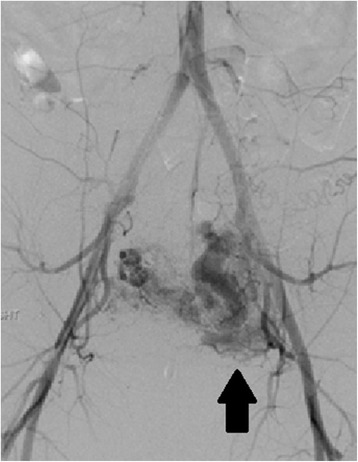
Left uterine artery arterio-venous malformation (AVM) by pelvic angiogram. This patient had undergone an uncomplicated ultrasound-guided D&C for a 10 week size cesarean scar ectopic pregnancy 2 months prior to presentation with vaginal bleeding and diagnosis of a left uterine artery AVM (arrow). The AVM was embolized with coils, but the patient required emergent hysterectomy for hemorrhage
